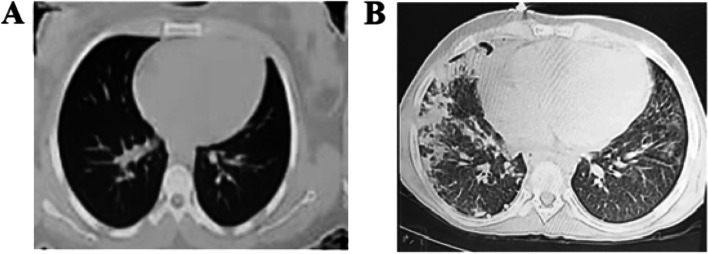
Chest CT of the patient. A At admission on the first presentation. B after 3 days of appearance of respiratory manifestations
